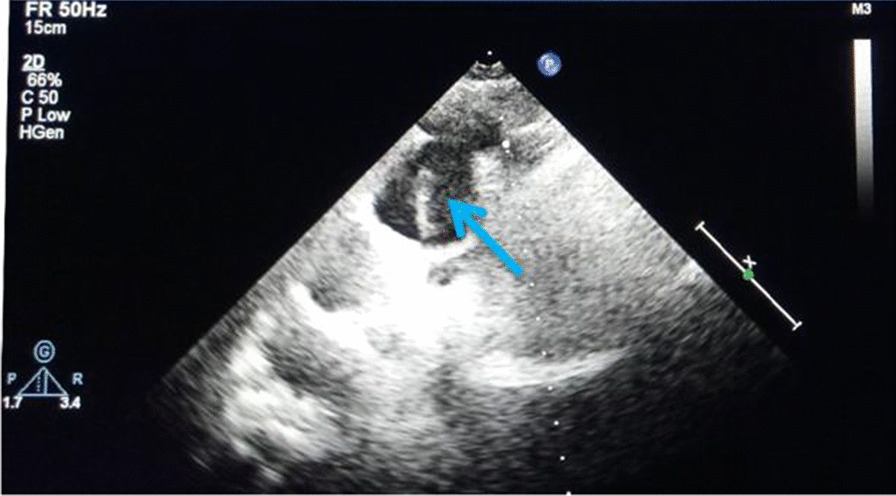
Echocardiographic examination in suprasternal notch long-axis view
